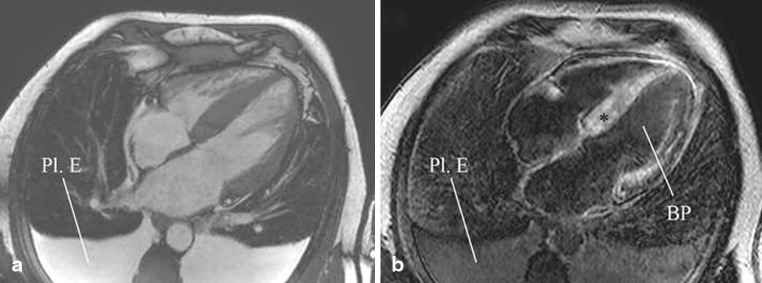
Cardiovascular magnetic resonance imaging: a Still frame of a cine horizontal long-axis view, showing ventricular hypertrophy and massive bilateral pleural effusion (*Pl. E*). b Delayed enhancement image in horizontal long-axis view obtained 10 min after intravenous gadolinium administration, showing a typical ‘cardiac amyloidosis pattern’: global myocardial enhancement (*) and a dark blood pool (*BP*)
